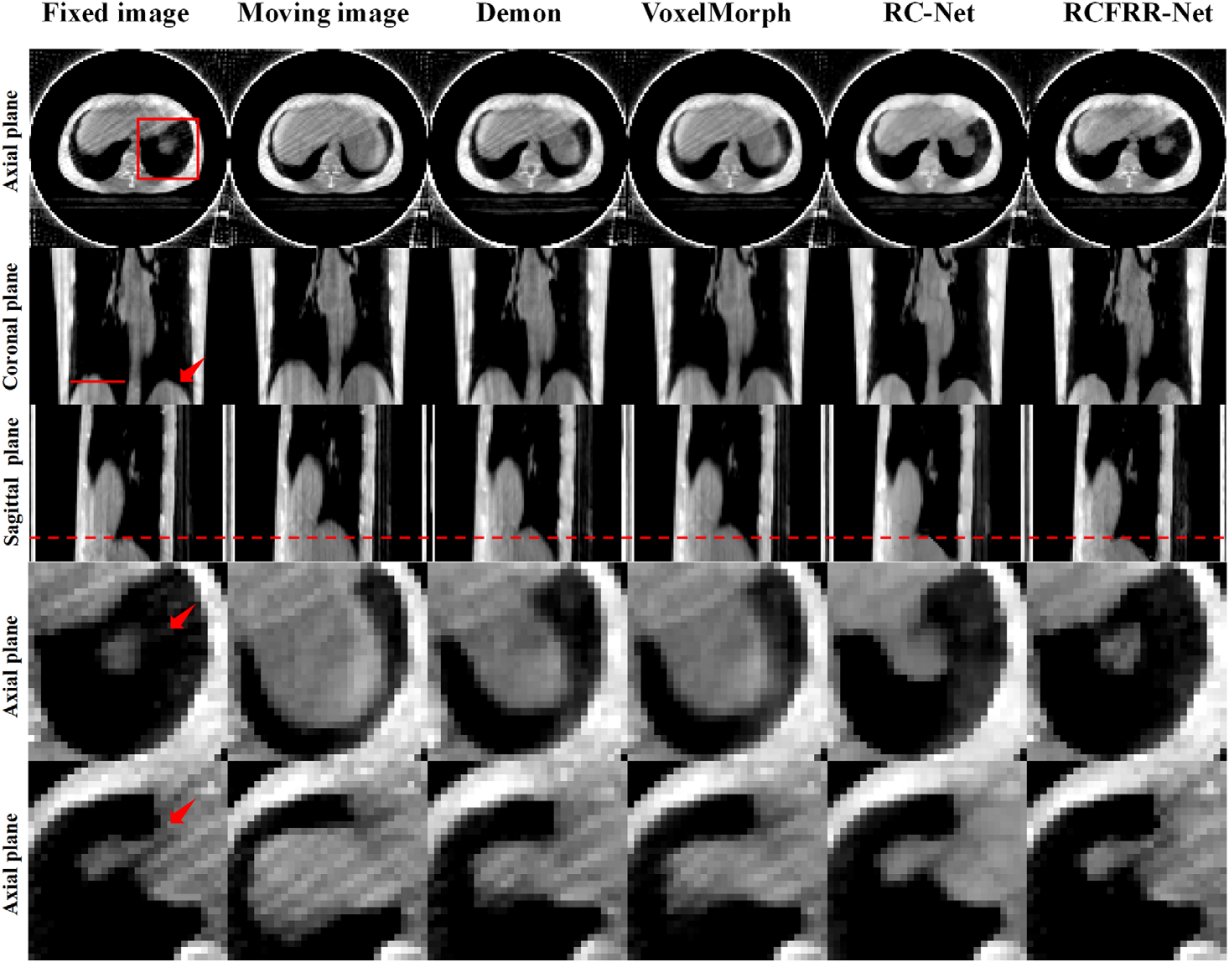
Comparison of the moving images, fixed images, and warped images of different registration methods on 4D‐CBCT datasets. The red dotted lines indicated the organs with large motion. Display window: [−800, 200] HU.
